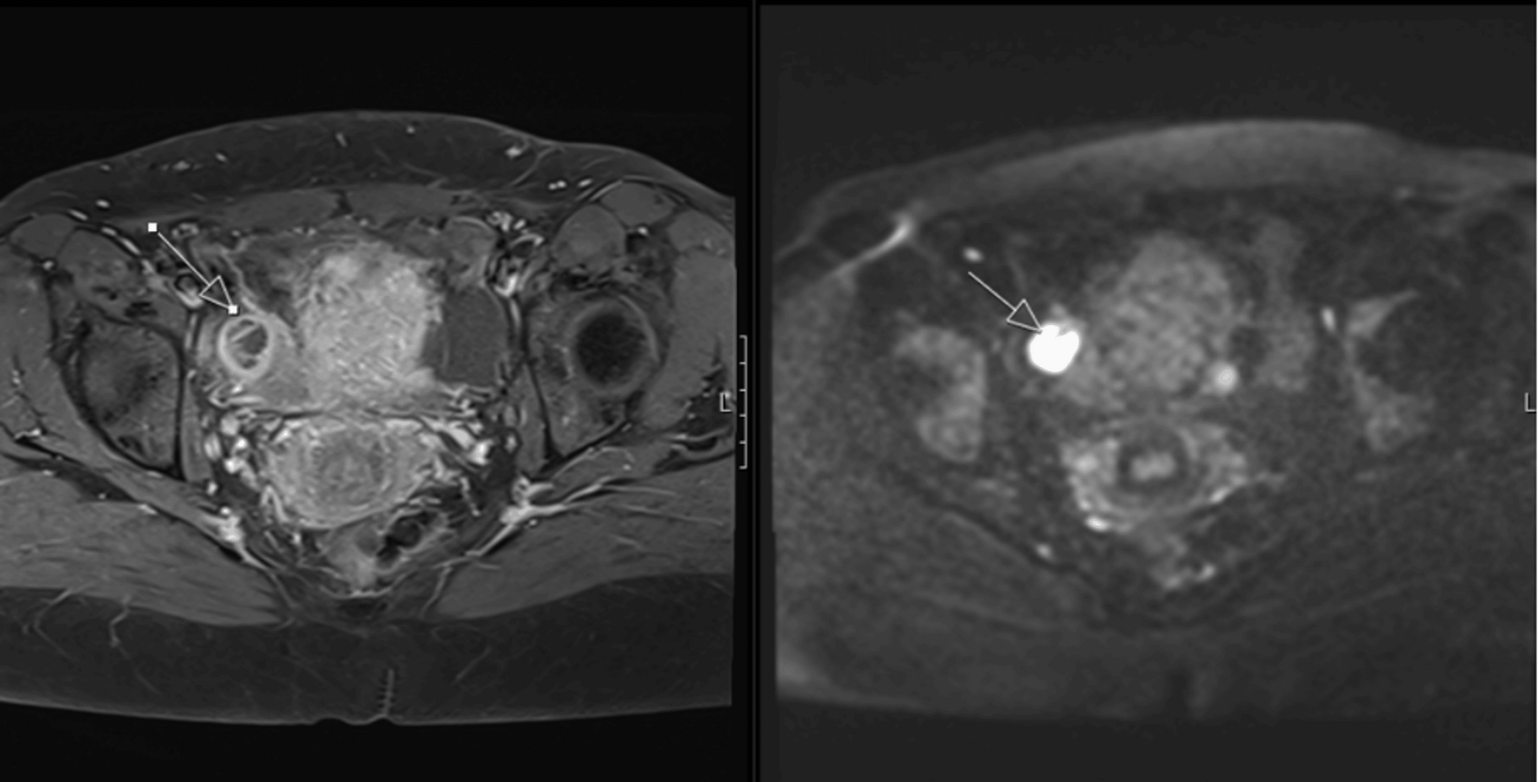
Follow-Up MRI Pelvis Showing Enhancing Adnexal Nodules Post-contrast MRI pelvis demonstrating enhancing soft-tissue nodules in adnexa (arrow) with restricted diffusion, correlating with FDG-avid lesions seen on PET/CT. Post-contrast T1-weighted image (left) and diffusion-weighted image (right). FDG: fluorodeoxyglucose; PET: positron emission tomography.
